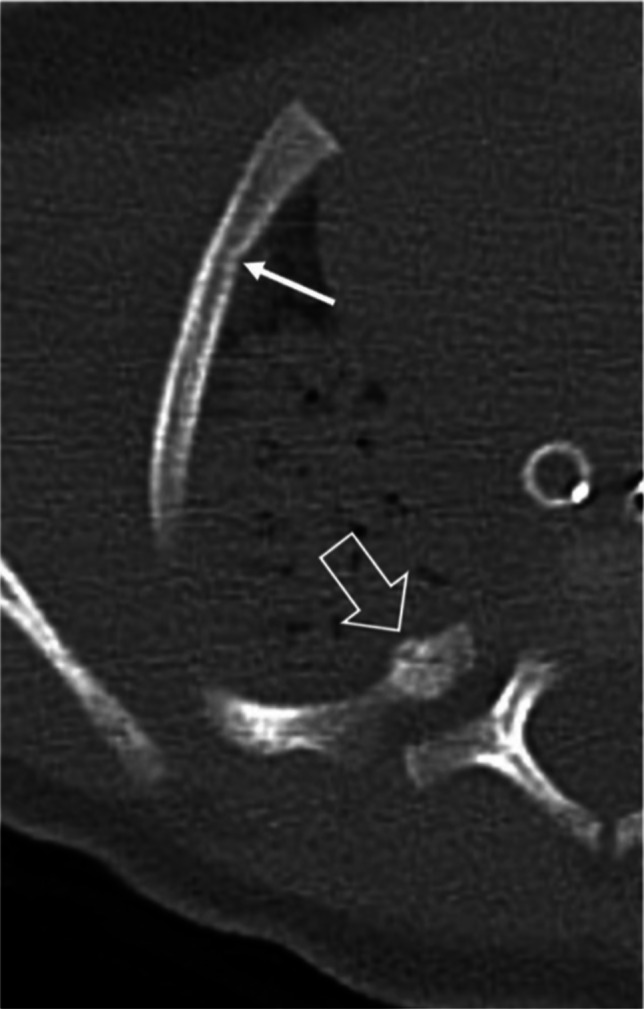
Axial postmortem CT of the chest in a 60-day-old boy following suspected physical abuse. High-dose acquisition and sharp reconstruction kernels allow high-quality imaging demonstrating in this case buckle deformity of the anterior rib (*closed arrow*) likely related to resuscitation, and a healing posterior rib fracture (*open arrow*), consistent with physical abuse. Reproduced with permission [34]
